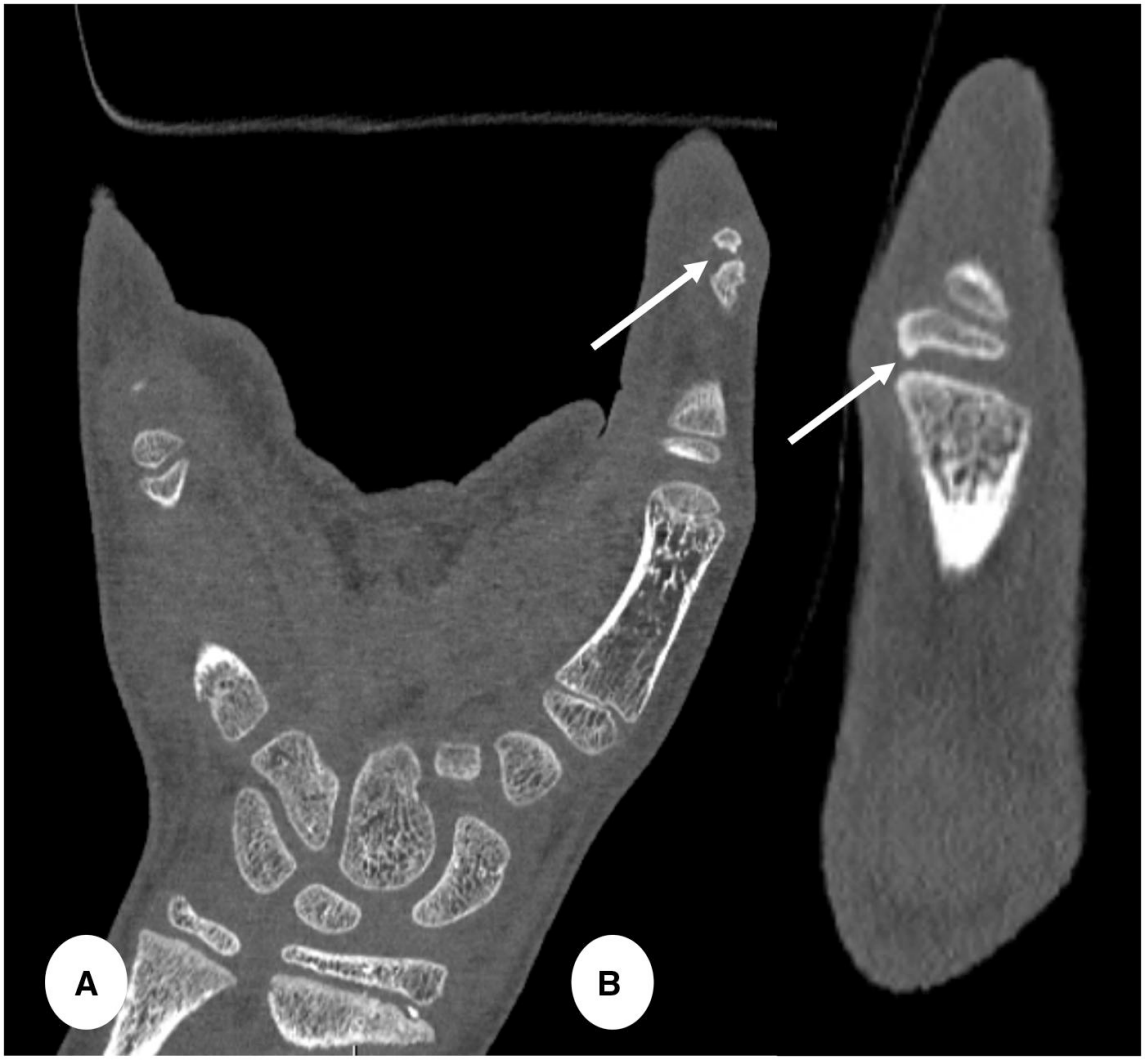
Hand CT in 10 years old male with swelling and pain proximal interphalangeal (PIP) joint of the left thumb from Trevor disease. (A) Coronal and (B) magnified sagittal PCD-CT images (slice thickness 0.2 mm, reconstruction kernel Br89 and IR level 3, Q3) showing small exophytic bone apposition (arrows in A and B) at the level of the proximal epiphysis of the PIP joint of the left thumb, likely representing small osteochondroma.
